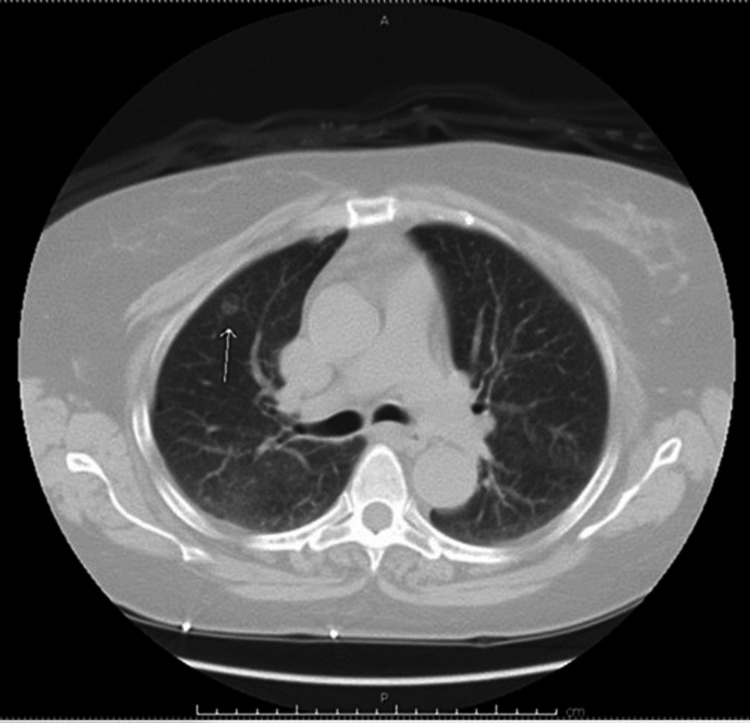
CT of the chest without contrast showing right upper lobe nodule (white arrow). CT: computed tomography
